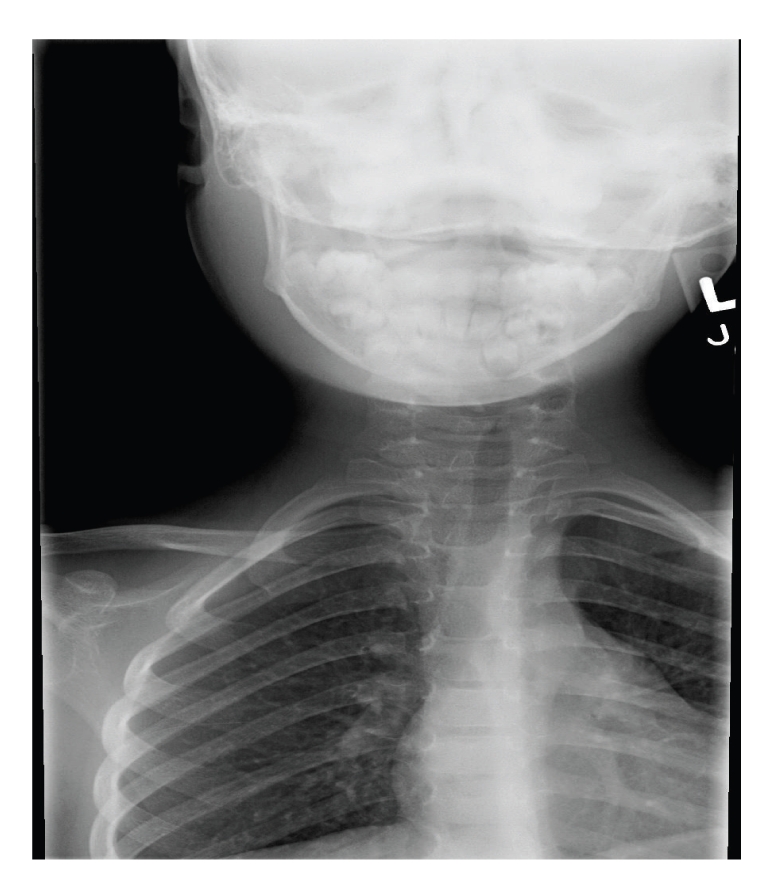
Soft tissue radiograph of the neck with deviation to the left and narrowing of upper airway.
